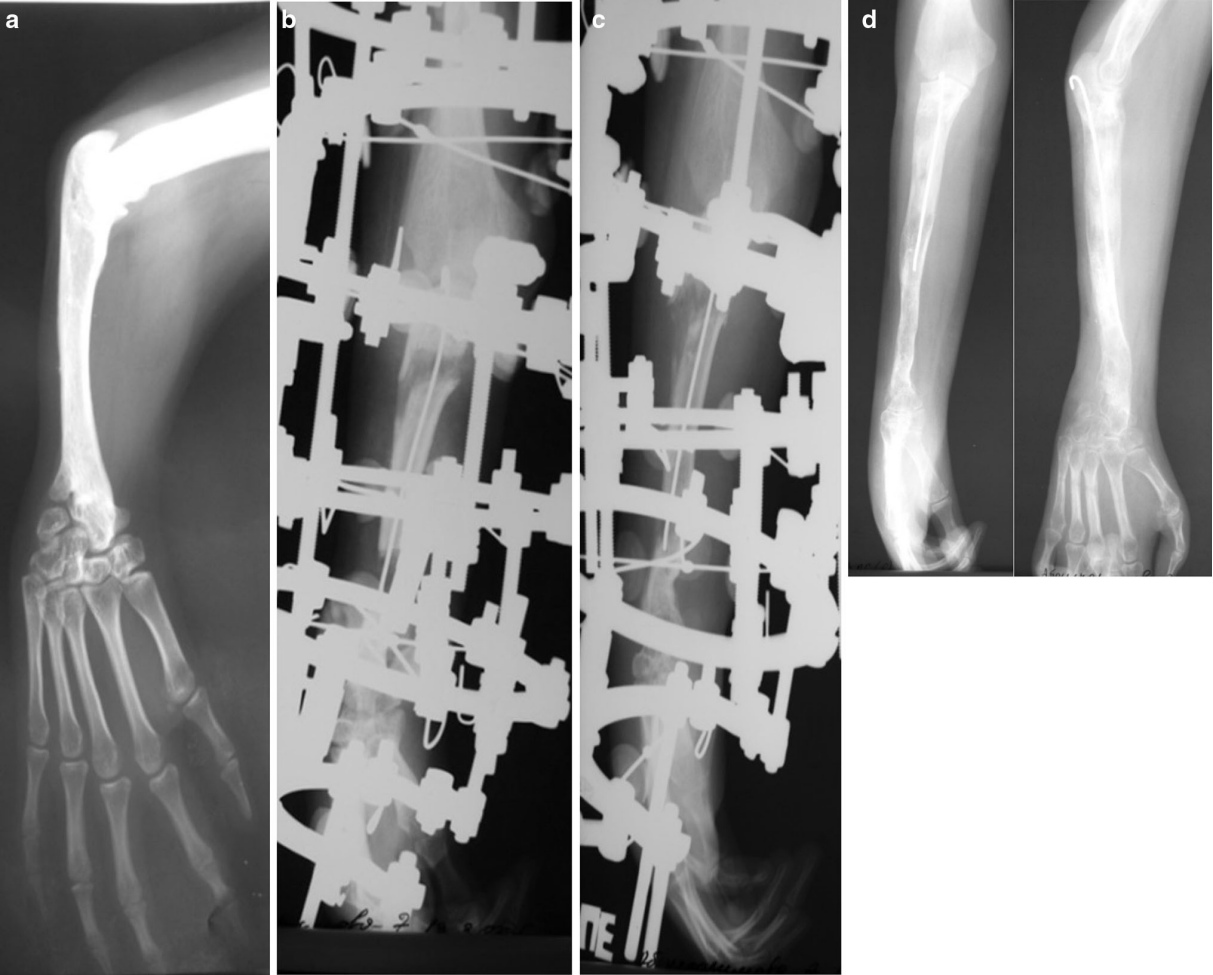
Example of forearm lengthening: a X-ray image of forearm before treatment; b premature bone consolidation noticed; c distraction is restarted after an unscheduled osteotomy; d X-ray image after frame removal with planned gain in length achievedintramedullary nails become blocked against the wires or half-pins of the external fixator;difficulty in maintaining an appropriate rate of distraction, when only one of the two forearm bones is fixed with a nail;the external fixator is removed before the adequate bone consolidation—is essential to understand that FIN is not a stand-alone osteosynthesis procedure and it cannot ensure that there will be no secondary fractures or deformities when the quality of the regenerated bone is insufficient to allow the removal of the external fixator;osteomyelitis related to FIN.
